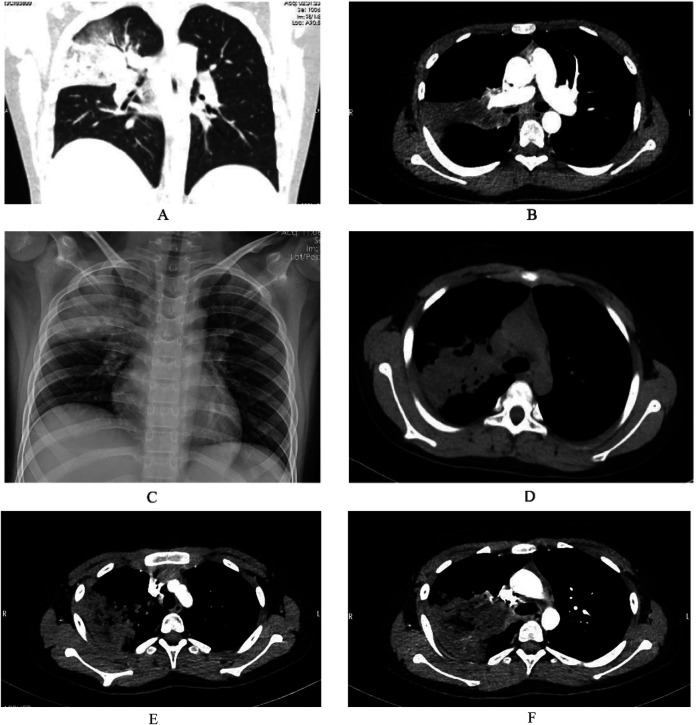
Partial consolidation in the right upper lobe, subpleural nodular lesions in the basal segments of both lower lobes, stenosis at the origin of the right upper lobe bronchus, and multiple enlarged lymph nodes in the mediastinum and right hilum (A). Pneumonia in the right upper lobe with partial consolidation, containing irregular lower-density areas, no significant enhancement on contrast scanning, stenosis at the origin of the bronchus in the right upper lobe, localized air trapping, and reduced branches of the right upper lobe pulmonary artery. There is no contrast filling in the distal right upper lobe pulmonary artery (B). A large confluent consolidation in the upper right lung field with uneven density compared to the previous study (C). Compared with the previous CT, the patchy and consolidated areas in the right upper lobe have decreased in size, with new circular and irregular cavitary formations, slightly increased density, new nodules, and faint patchy shadows in the right lower lobe. Subpleural nodules are noted in the outer basal segments of both lower lobes, and the local narrowing of the right mainstem bronchus remains unchanged as before (D). Reduced branching of the right upper lobe artery, distal filling defects in the posterior segmental pulmonary artery of the right upper lobe (E), and vascular destruction changes (F).
